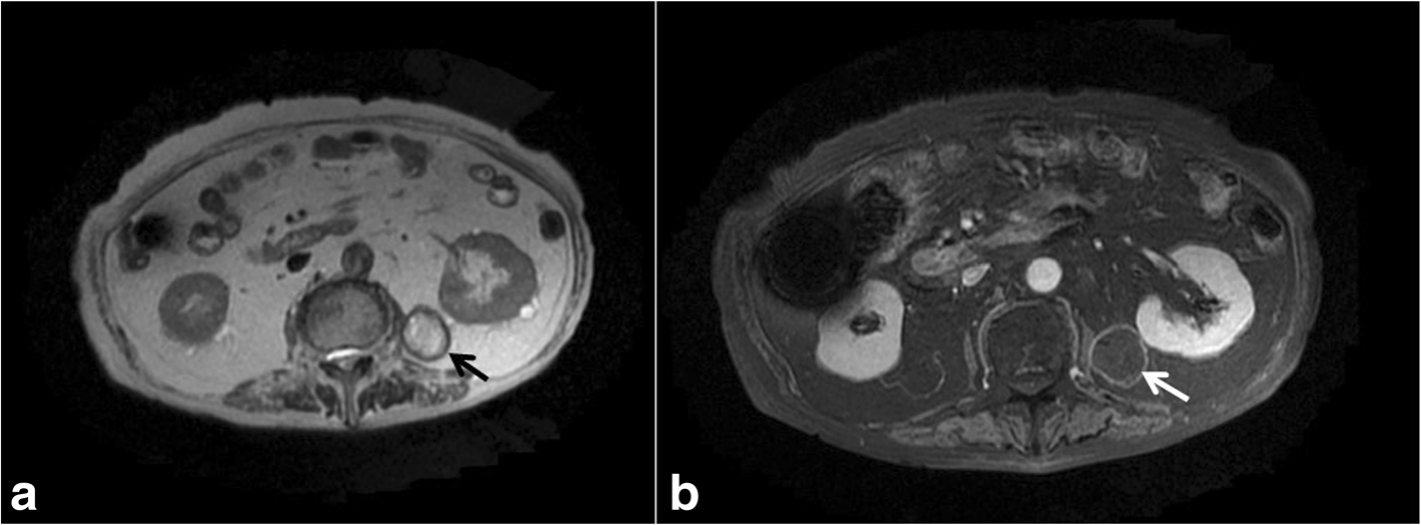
MRI of the abdomen and pelvis: a axial T2. b Fat suppression axial T1 images. The mixed heterogeneous signal of the high-low signal in both the T2 and T1-weighted images, and signal strength, decreased with T1 fat suppression
